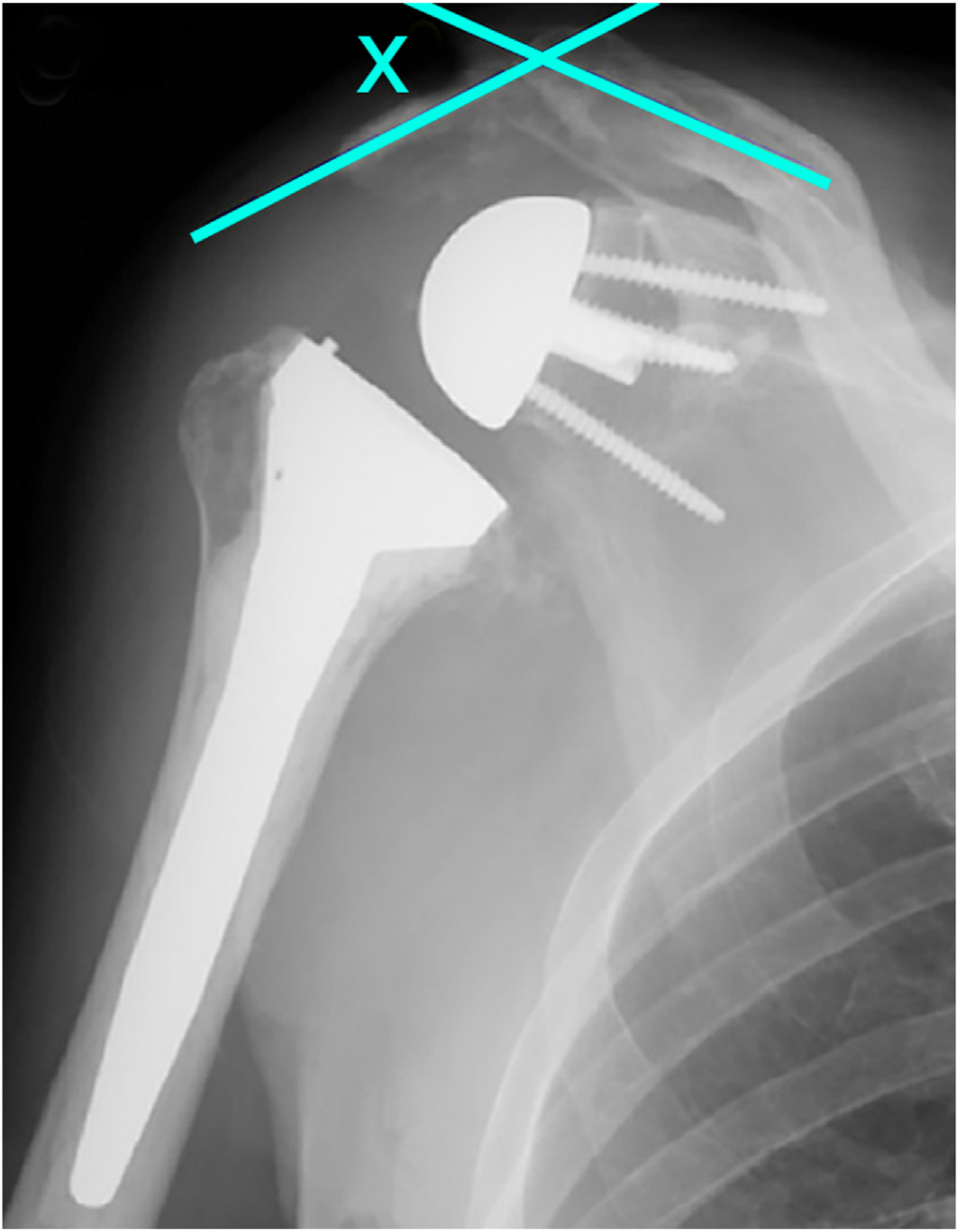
Inferior acromial tilting is measured on anteroposterior (AP) radiographs as the angle between the distal os segment and the proximal acromion. Aibinder et al has described tilting as any angle >5°.^2^
